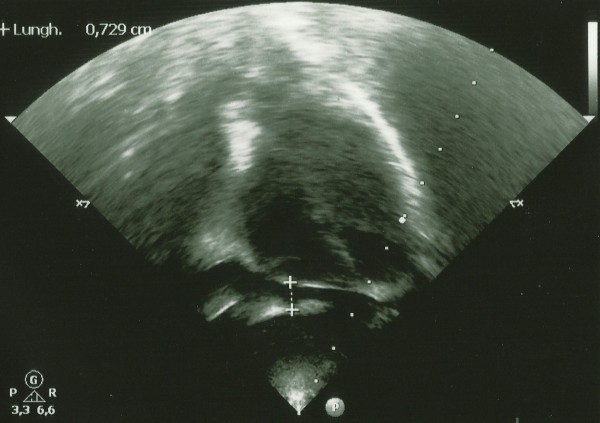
Echocardiographic findings: pericardial effusion and perivascular brightness of the proximal left descending coronary artery.
